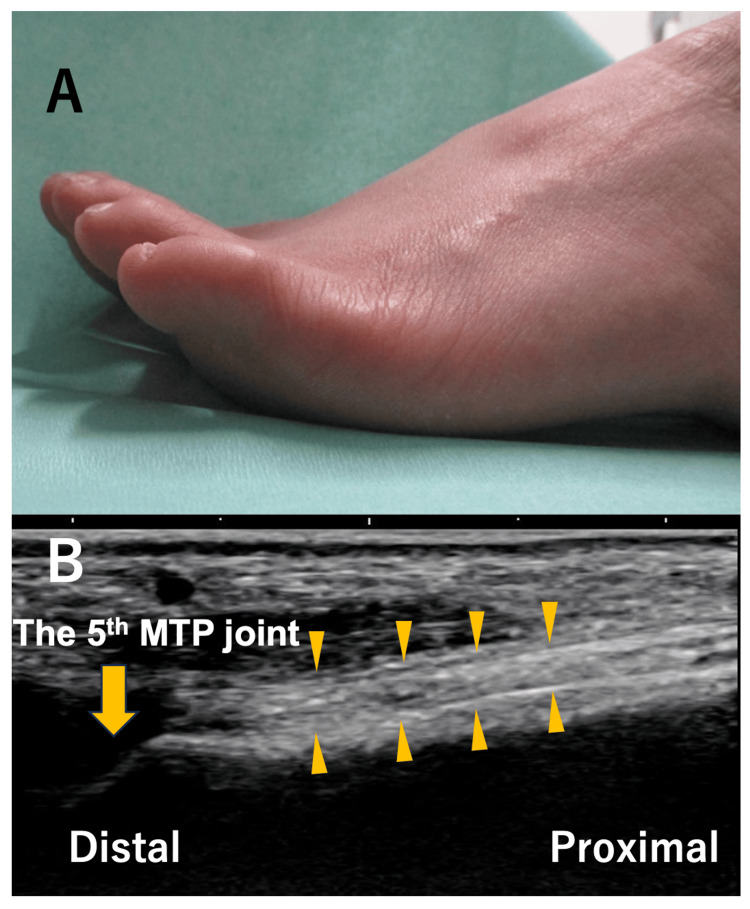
One year postoperatively, (A) extension of the left fifth toe MTP joint was restored, and (B) ultrasonographic evaluation revealed no abnormalities in the extensor tendon contour. The arrowheads indicate the extensor digitorum longus tendon MTP: metatarsophalangeal
